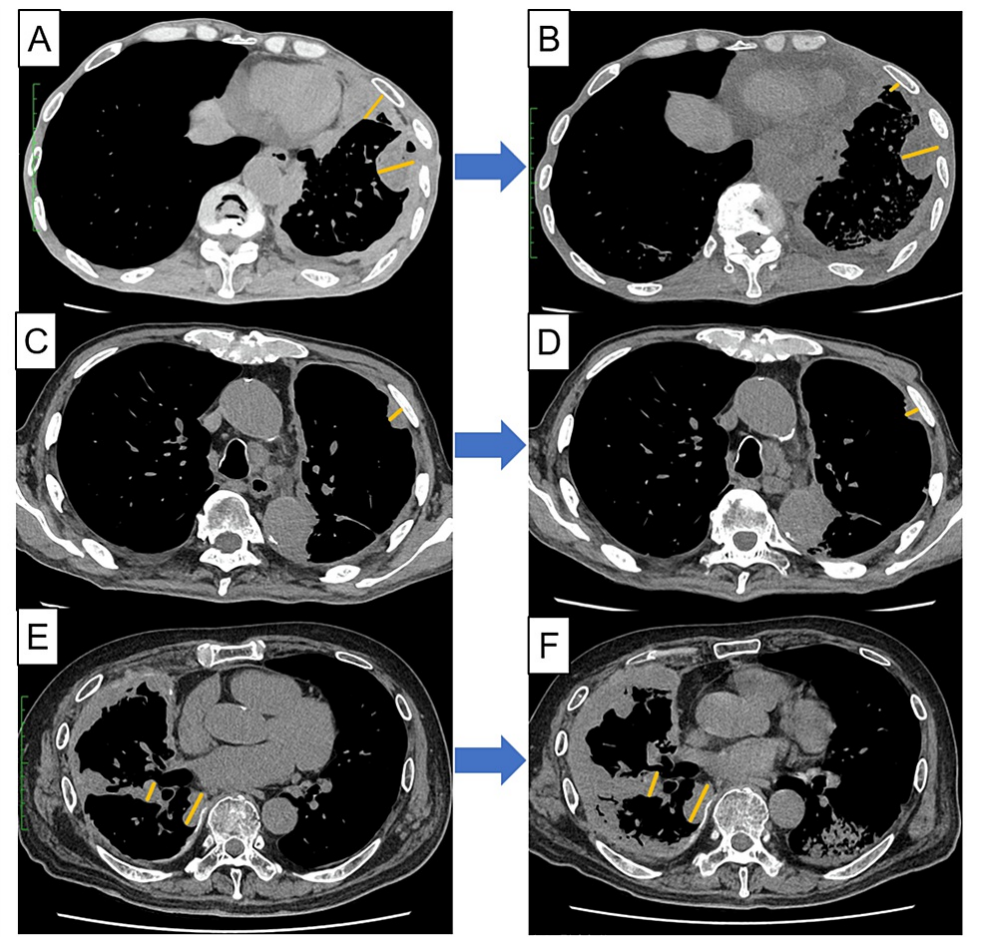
First CT evaluation during treatment with nivolumab plus ipilimumab The CT scan shows a size reduction in lung cancer in the first three cases. (A) A chest CT at the start of treatment in Case 1; (B) A chest CT after 2.5 months from treatment initiation; (C) A chest CT at the start of treatment in Case 2; (D) A chest CT after 1.8 months from treatment initiation (E); A chest CT at the start of treatment in Case 3; (F) A chest CT after 1.8 months from treatment initiation. Yellow lines indicate target lesions.
